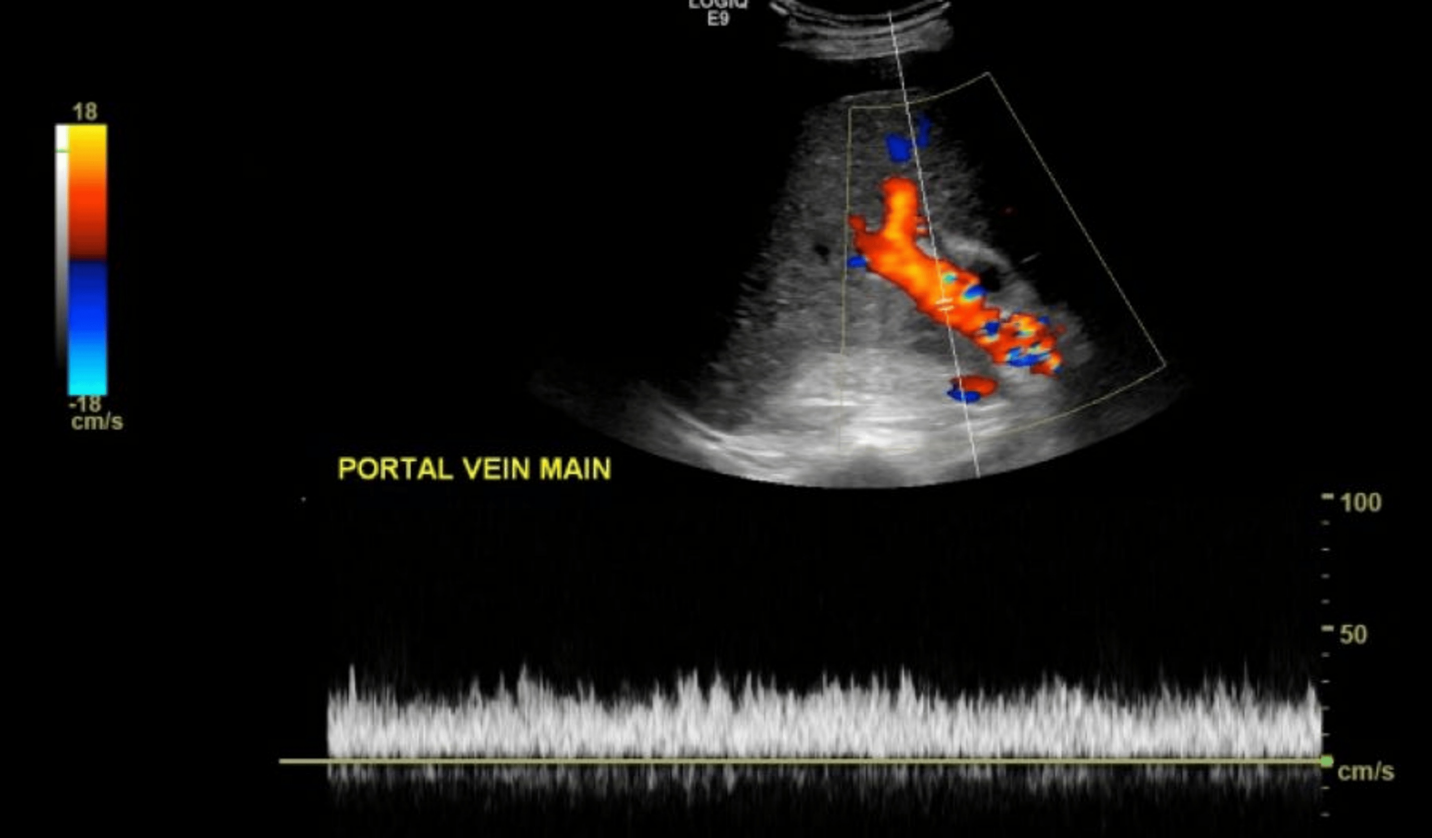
Doppler ultrasound of the main portal vein demonstrating patent vessel with normal hepatopetal flow
